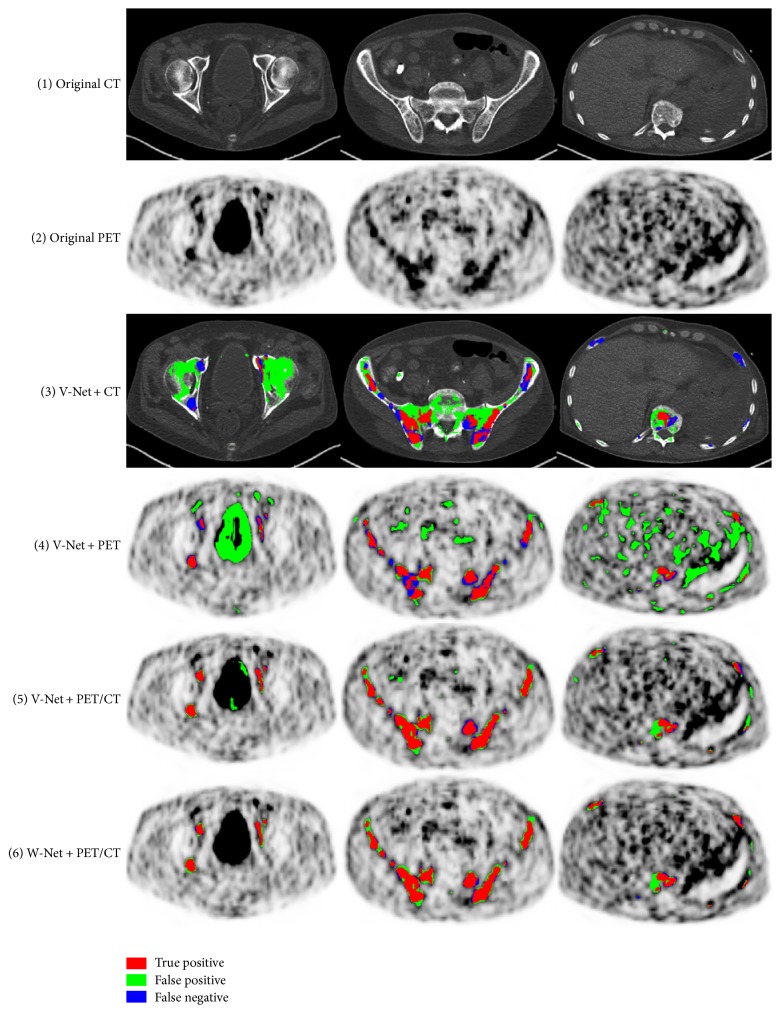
Exemplary detection results of V-Nets and W-Net: (1) the original axial CT slices; (2) the corresponding PET slices; (3) MM lesion prediction using CT alone in V-Net; (4) MM lesion prediction using PET alone in V-Net; (5) MM lesion prediction using PET/CT in V-Net; (6) MM lesion detection using W-Net.
